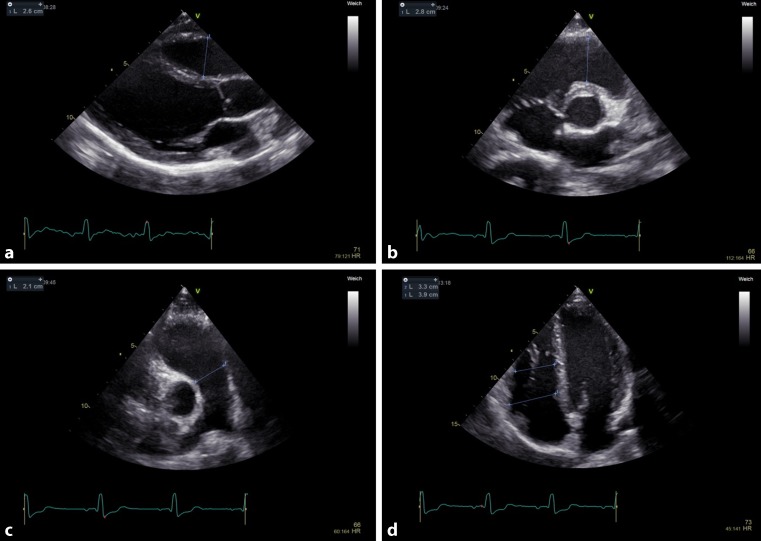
Systematic evaluation of right ventricular size from four standardized transthoracic views and their normal values. a Proximal outflow tract parasternal long axis view; >30 mm abnormal, normal range 20–30 mm. b Proximal outflow tract parasternal short axis view; >35 mm abnormal, normal range 21–35 mm. c Distal outflow tract; >27 mm abnormal, normal range 17–27 mm. d Apical right ventricle at base; abnormal: >41 mm, normal range 25–41 mm. Apical right ventricle at mid-level; abnormal: >35 mm, normal range 19–35
